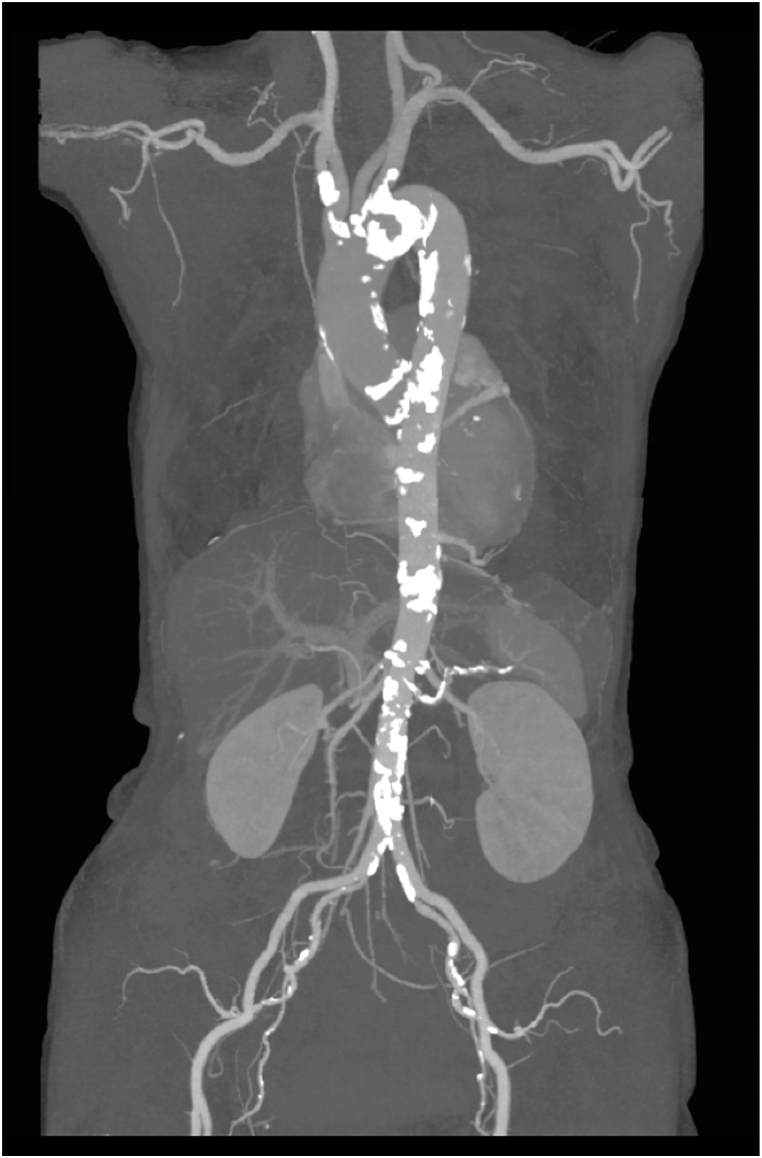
Preoperative computed tomography image of the aorta.
